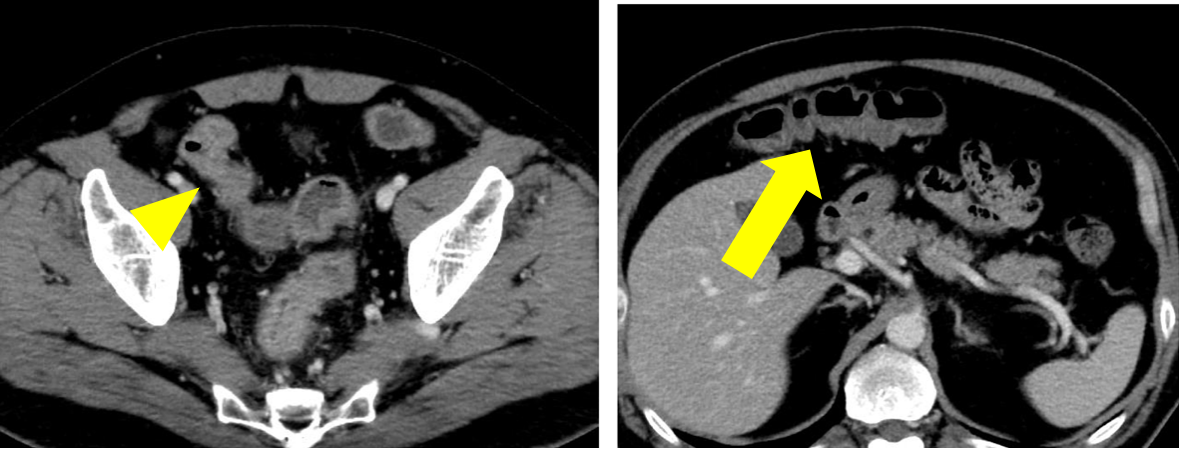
First abdominal CT scans. CT revealing edematous thickening bowel from the rectum to the transverse colon (arrow). The appearance of the cecum is normal (arrowhead)
